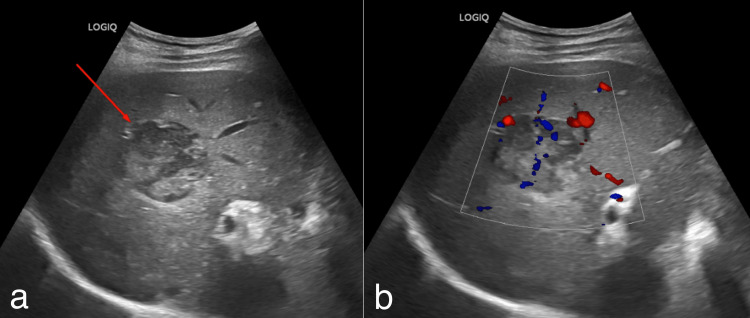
Ultrasound of the abdomen. A few relatively well-defined heteroechoic lesions are noted in the right lobe of the liver, indicated by the red arrow (a), showing mild internal and peripheral vascularity on color Doppler (b).
